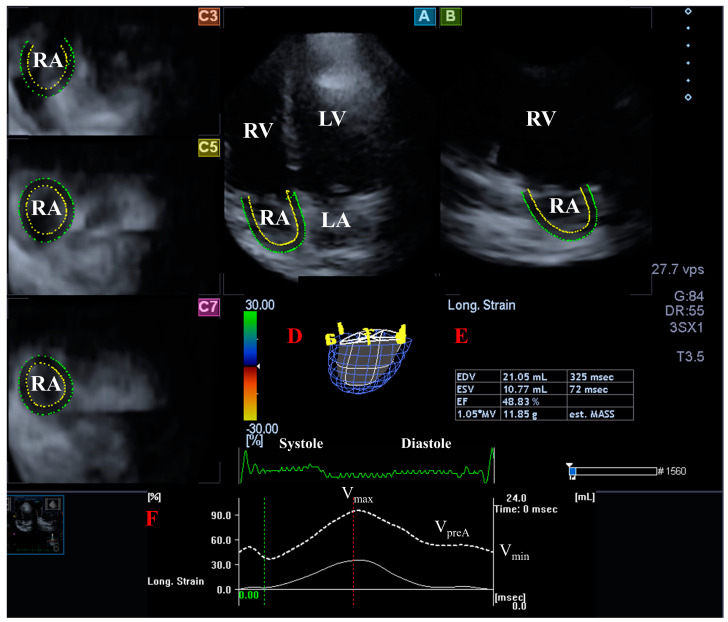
Assessment of the right atrium (RA) by three-dimensional (3D) speckle-tracking echocardiography: apical longitudinal four-chamber (A) and two-chamber views (B), and 3 short-axis views at basal (C3), midatrial (C5), and superior (C7) RA levels. Virtual 3D RA cast (D), RA volumetric data (E), time-global RA volume change curve (dashed white curve) and time-global RA longitudinal strain curve (white curve) respecting the cardiac cycle are also shown (F). Abbreviations. LA = left atrium, LV = left ventricle, RA = right atrium, RV = right ventricle, EDV = end-diastolic volume, ESV = end-systolic volume, EF = ejection fraction, est. = estimated, MV = myocardial volume. V_max_ = maximum right atrial volume, V_preA_ = volume at the onset of atrial systole, V_min_ = minimum right atrial volume.
